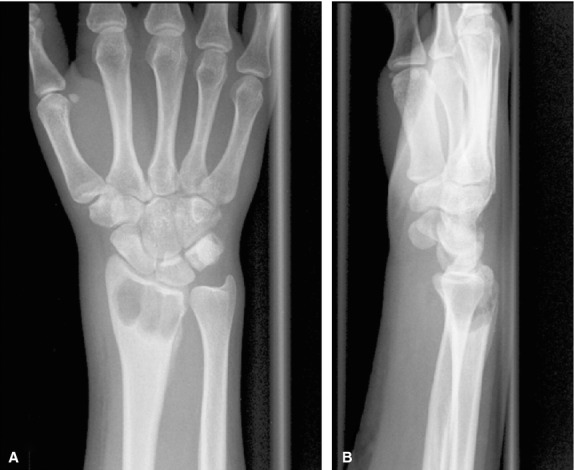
Pre-operative X-ray of the wrist (case 2) showing cystic formations at the distal radius. (A) Posteroanterior radiograph. (B) Lateral radiograph.
